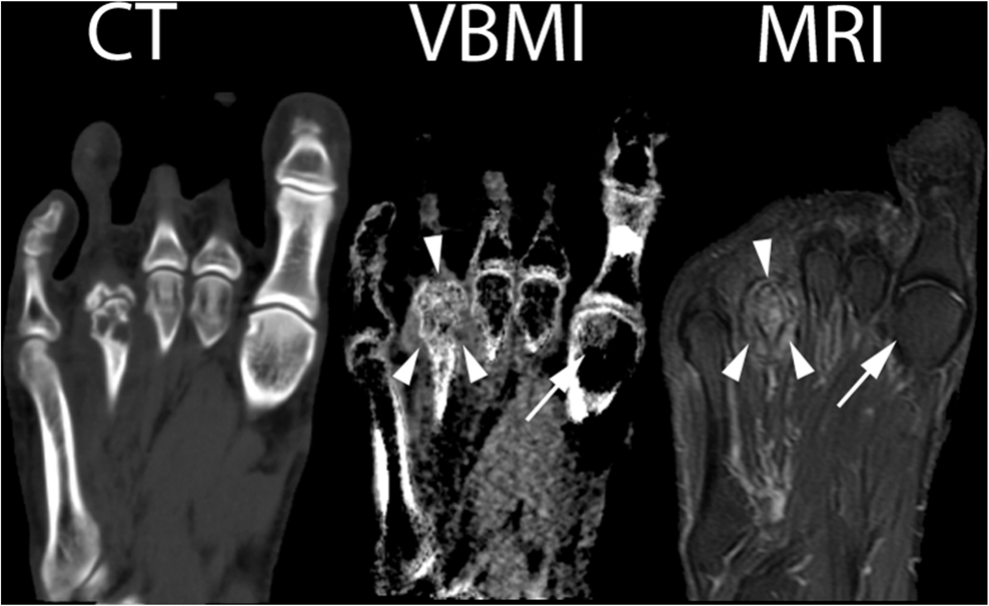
Right foot of patient No. 2 (seropositive RA). CT: normal CT image at 135 kV, VBMI: corresponding VBMI image, MRI: corresponding fat-saturated T2 sequence – VBMI displays the BME in the fourth metatarsal head (*arrowheads*) with erosions and cysts in CT. In the first metatarsal head, VBMI is false positive compared to MRI (*arrow*)
